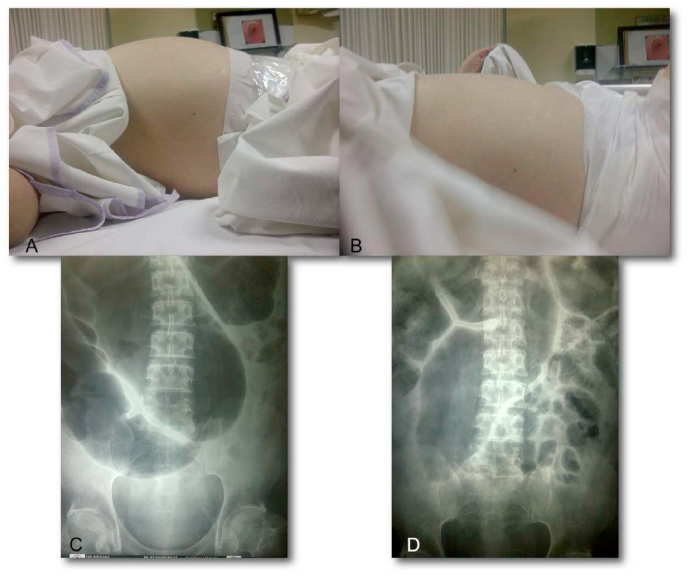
(A) Clinical condition before ileus tube placement. (B) Seven days after colonic decompression. (C&D) Plain abdominal X-ray of the distended large bowel and an air-fluid level.
